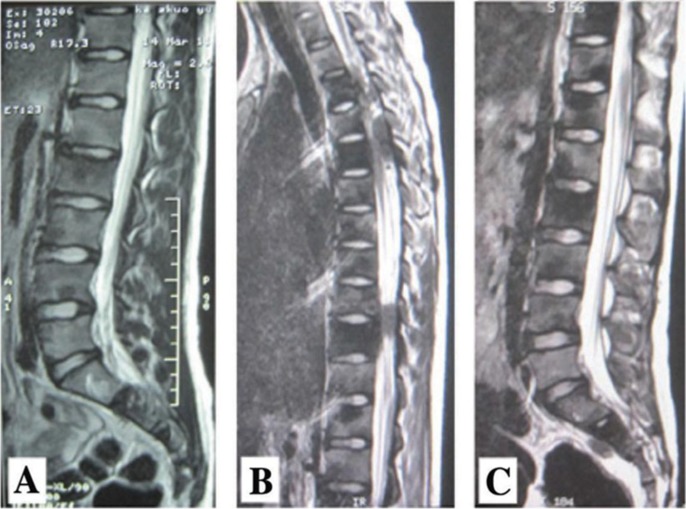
Thoracolumbar MRI (A) shows a short signal on T2-weighted MRI in the T2 to T11, L1 to L5, and sacral vertebra. (B, C) Flake low signal shadows are observed outside of the spinal cord and dura in the T4-T5 and T9-T10 spinal body plane. MRI taken after neoadjuvant chemotherapy (A) indicated that the number of lesions increased and the tumors in the spinal canal were amplified, as compared to that taken before treatment (B, C).
